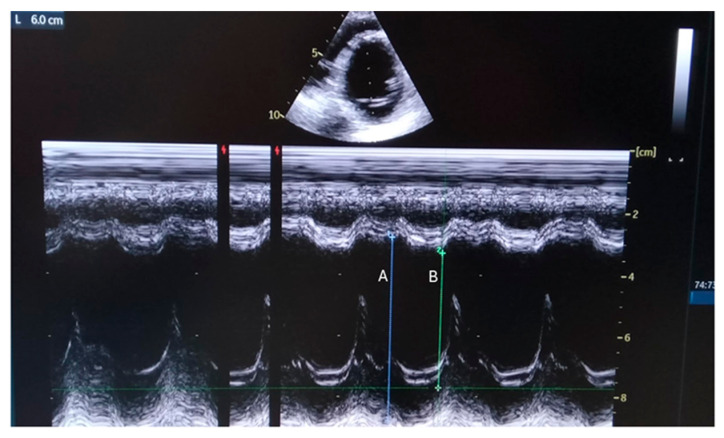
Parasternal M-mode view shows internal dimensions of dilated left ventricle with reduced ejection fraction (EF about 35%). A: End Diastolic Diameter=60 mm; B: End Systolic Diameter=44 mm, Fractional Shortening = 26%).
